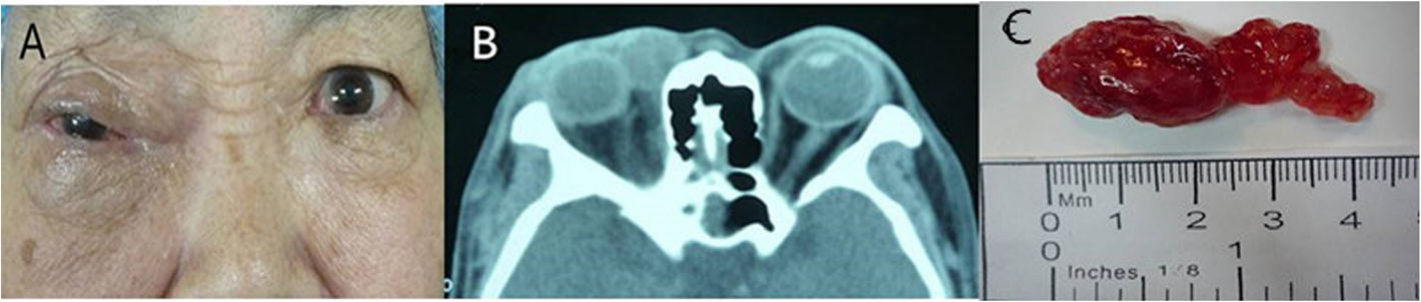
a Photograph showing swelling and ptosis of the right eyelid. b Contrast-enhanced orbital CT showing a well-defined mass which compressed the lateral eyeball. c Gross appearance of the tumor showing a lobulated-shaped mass with local necrosis
